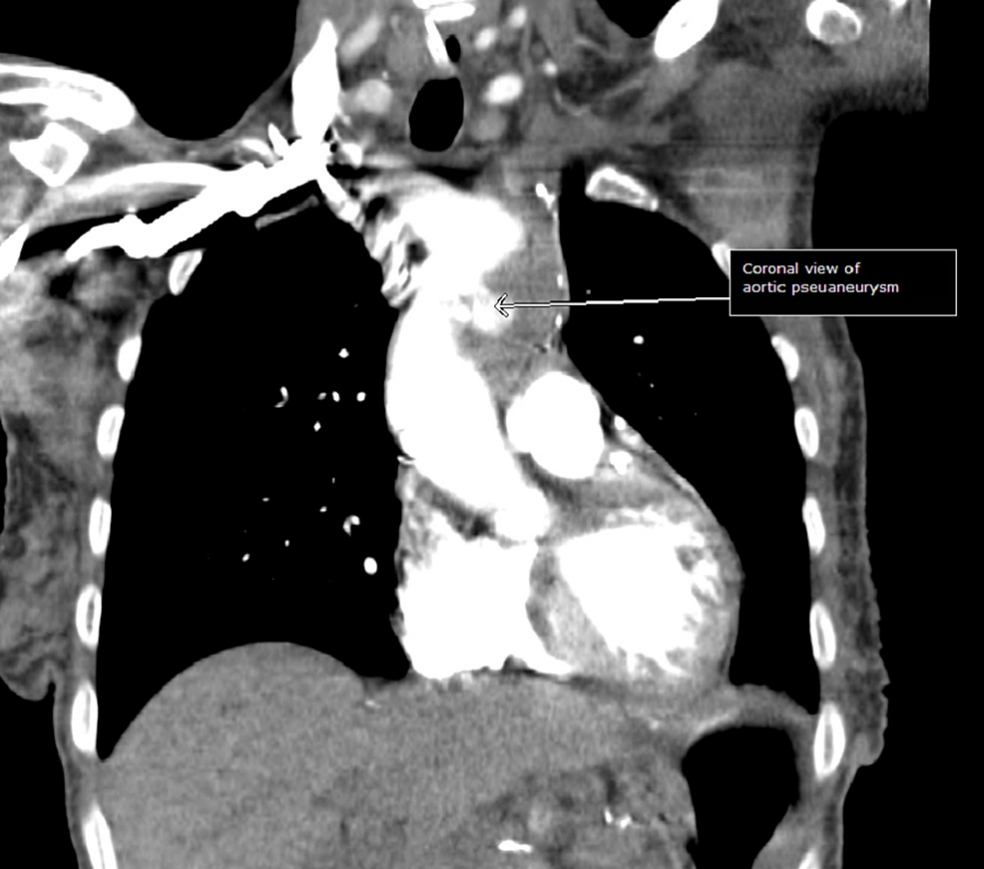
CT angiogram coronal view.
